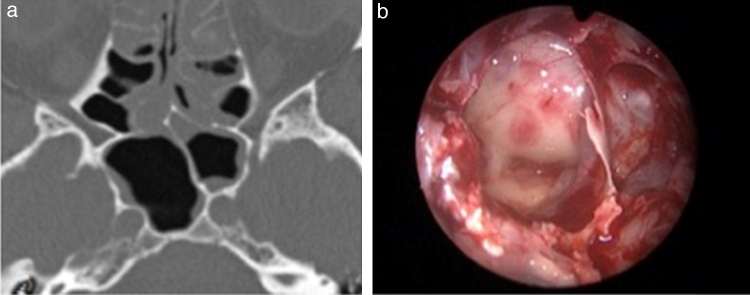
(a) CT scan axial view of an intersphenoid septation in the left internal carotid artery protuberance (ICAp) and (b) intraoperative view (0° endoscope) showing the same septation in the left ICAp.
